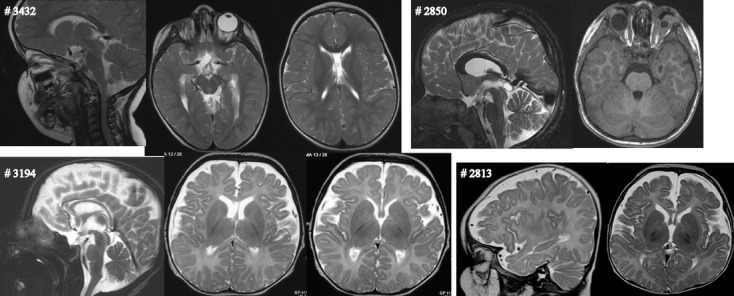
Cerebral abnormalities associated with a mutation in *SOX2* gene. Cavum vergae in a 3-year-old child with unilateral anophthalmia (CaseID 3432; proton-weighted sagittal and transversal spine echo 3 mm scan). Pineal cyst in a child with bilateral anophthalmia (note: orbital expander OU; CaseID 2850 at age 4.5 years; T2-weighted sagittal and T1-weighted transversal spine echo 3-mm scan). Small posterior part of the corpus callosum and septum pellucidum cyst in a child with bilateral anophthalmia (CaseID 3194 at age 3 months; T2-weighted sagittal and transversal spine echo 3-mm scan). Fronto-temporal cerebral volume reduction (T2-weighted native sagittal spine echo 2 mm and transversal 4-mm scan) in a 3-month-old boy with bilateral anophthalmia (CaseID 2813)
